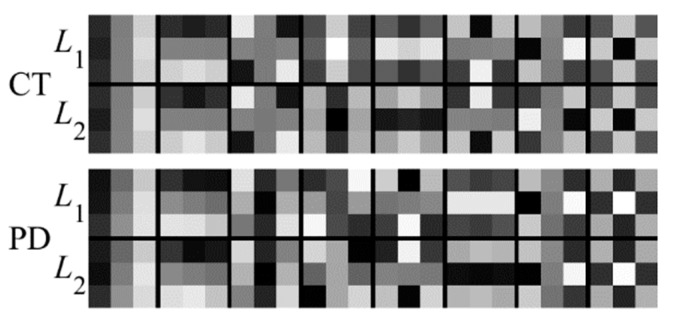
Filters learned for the multiple layers by the PCANet. The first and second rows are the filters at the first and second stages for the CT images, respectively; the third and fourth rows are the filters at the first and second stages for the PD images, respectively.
